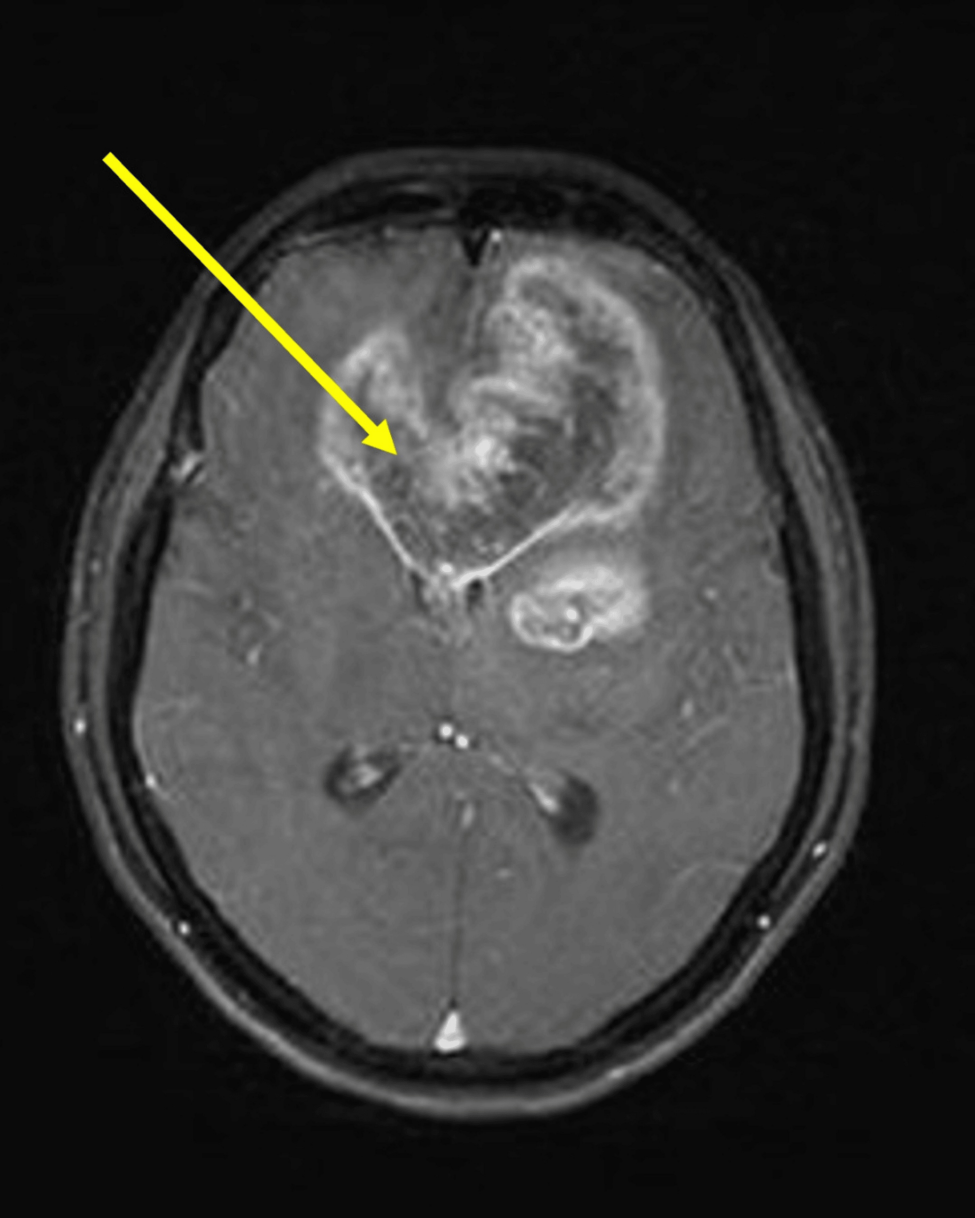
MRI brain showing the lesion with thick, irregular peripheral enhancement on T1 post-contrast imaging. These features are suggestive of glioblastoma.
